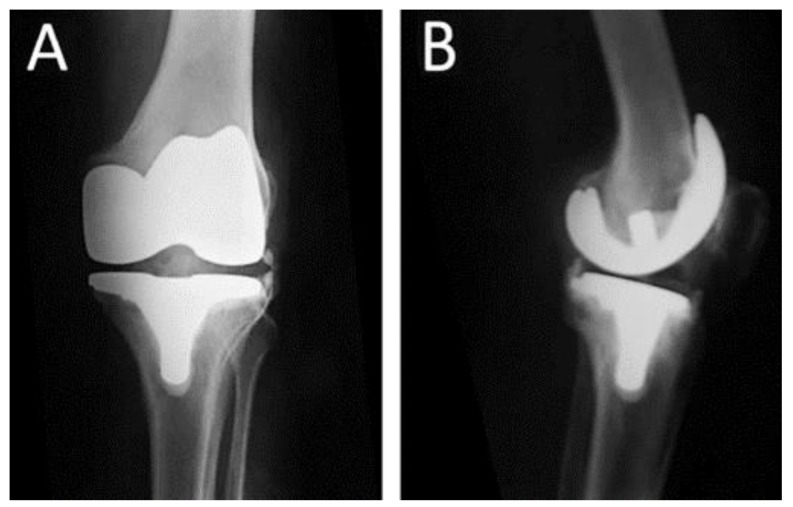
Postoperative radiographs of a 67-year-old female who underwent total knee replacement due to osteoarthritis of the left knee (A) Anteroposterior and (B). Lateral radiograph of the knee.
